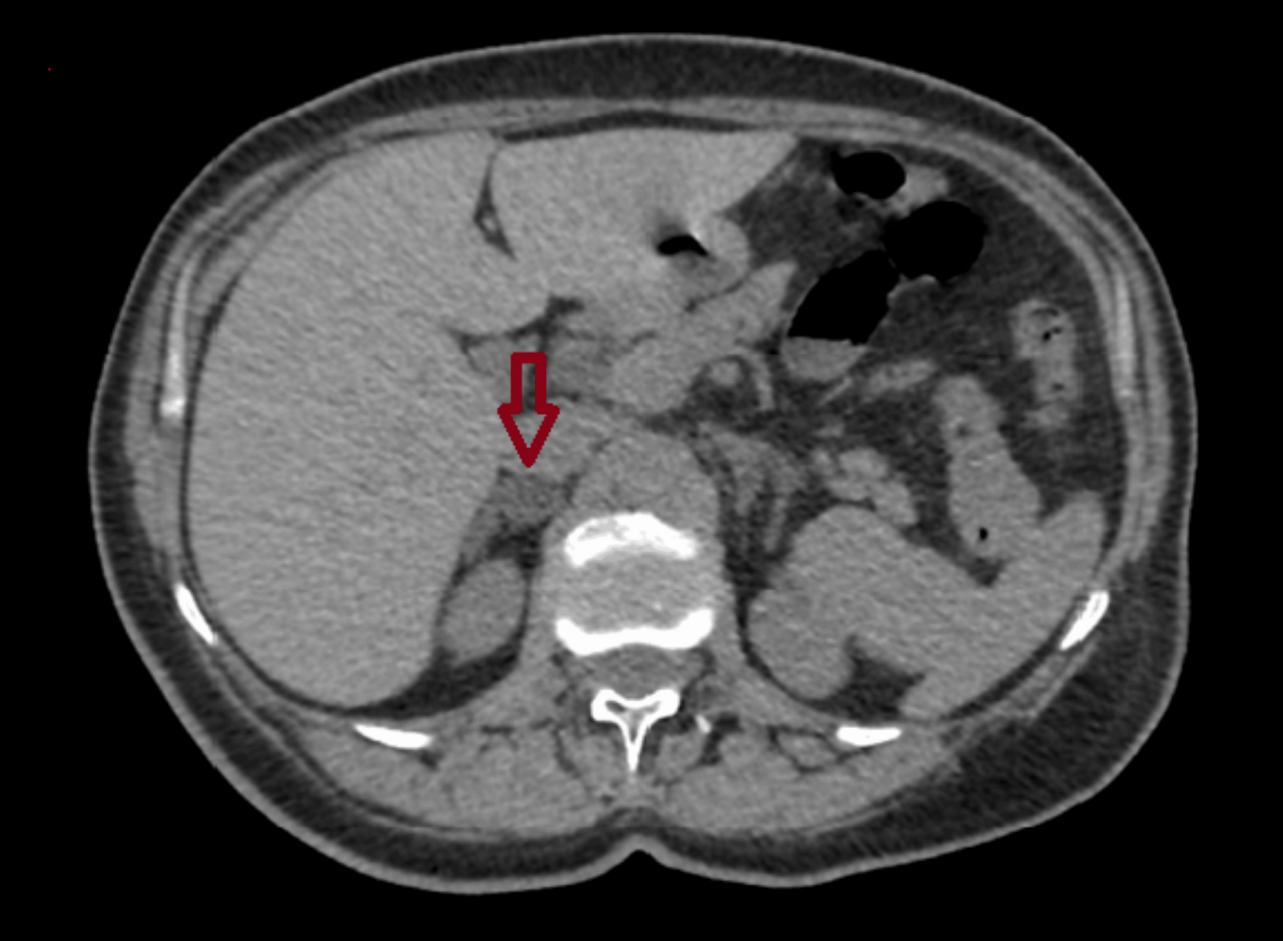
Adrenal imaging findings of Patient 1 An abdominal CT (transverse plane) of a 67-year-old woman showing a right adrenal incidentaloma measuring 18 × 15 mm.
